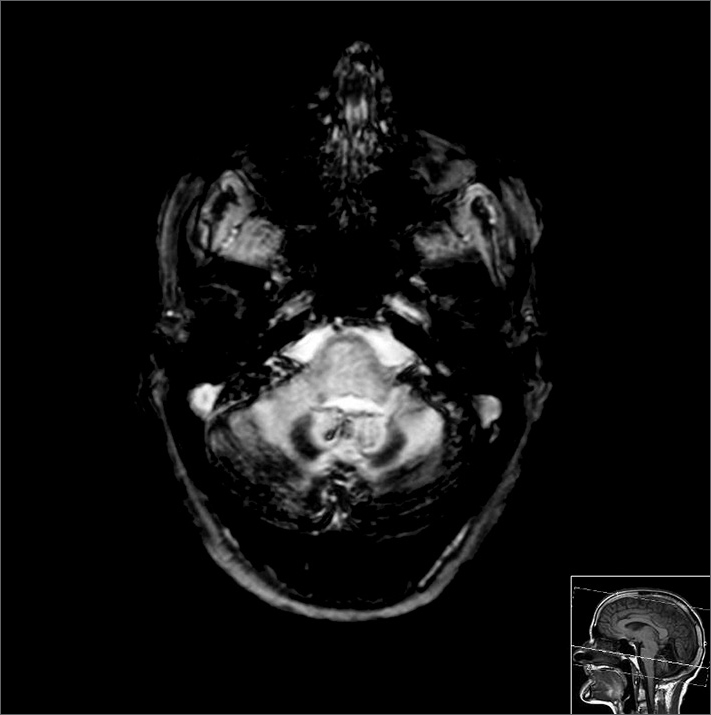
MRI - CE, axial slices in T2*, where we see intense hypo signal in T2* of the pial coating of all the structures in the posterior fossa, in the internal vertent of the occipital lobe and the Sylvian fissures, marked cerebellar atrophy, translating into superficial siderosis of the CNS.
